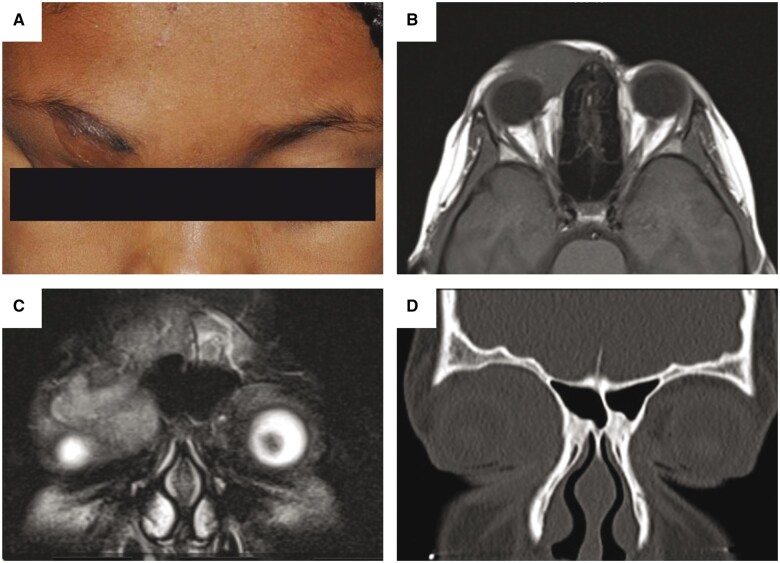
Clinical and radiographic features. A subcutaneous mass involving the superior nasal right periorbital region was noted on physical exam (A). Magnetic resonance imaging demonstrated the mass was primarily T1 isointense (B) and heterogeneously T2 minimally hypointense (C). Computerized tomography imaging demonstrated remodeling of the anterior wall of the right frontal sinus and orbital roof (D).
